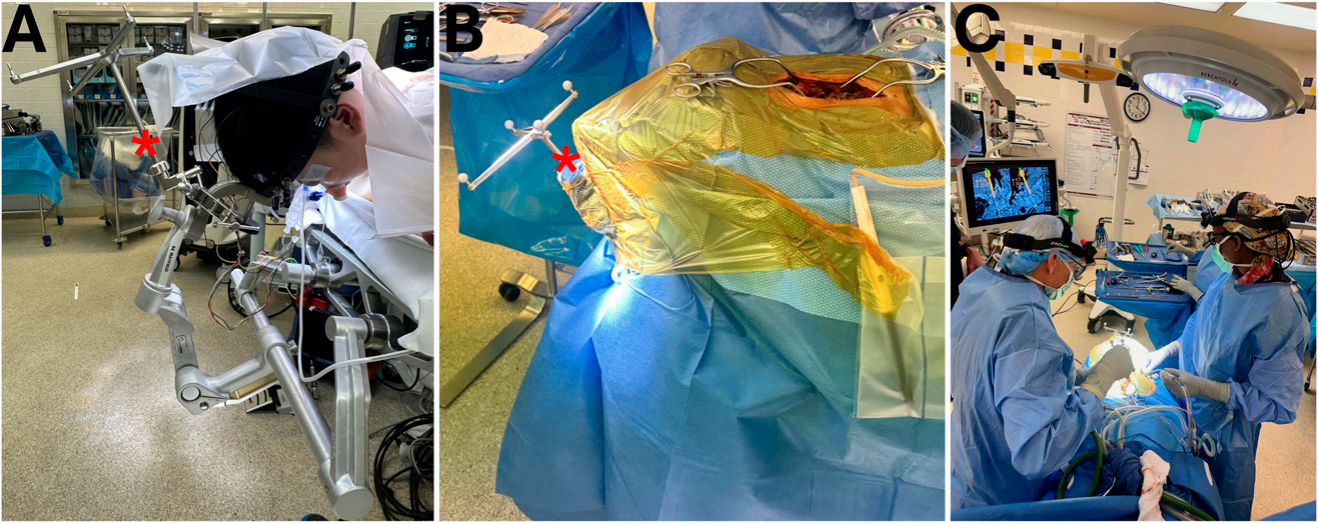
CT-guided navigation in a child with complex congenital cervical deformity. The reference array (*) is attached to the Mayfield cranial stabilization system (A), and sterile prepping and draping is performed around the array (B). The head of bed is turned 180 degrees away from anesthesia to facilitate intraoperative CT imaging and navigation. Intraoperative photo of thoracic pedicle screw (C) placement using CT navigation.
